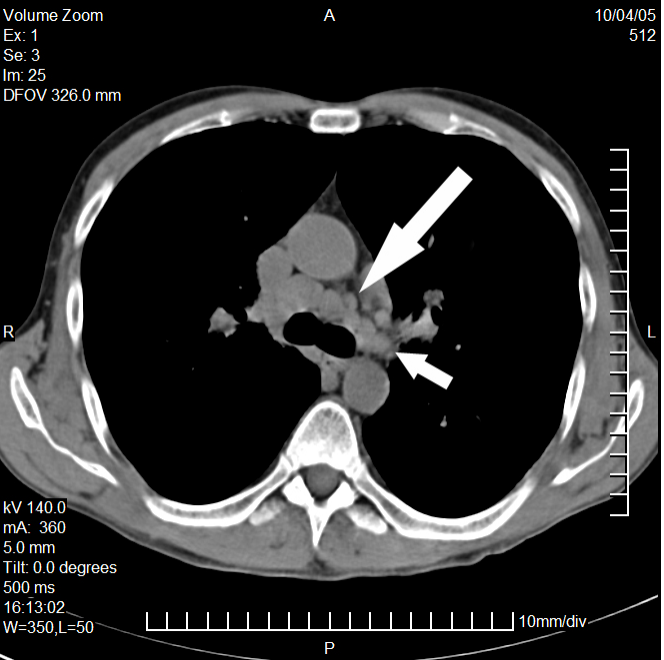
Computed tomography of the chest (I). CT of the chest: Distinct mediastinal (large arrow) and perihilar lymphadenopathy (small arrow)
